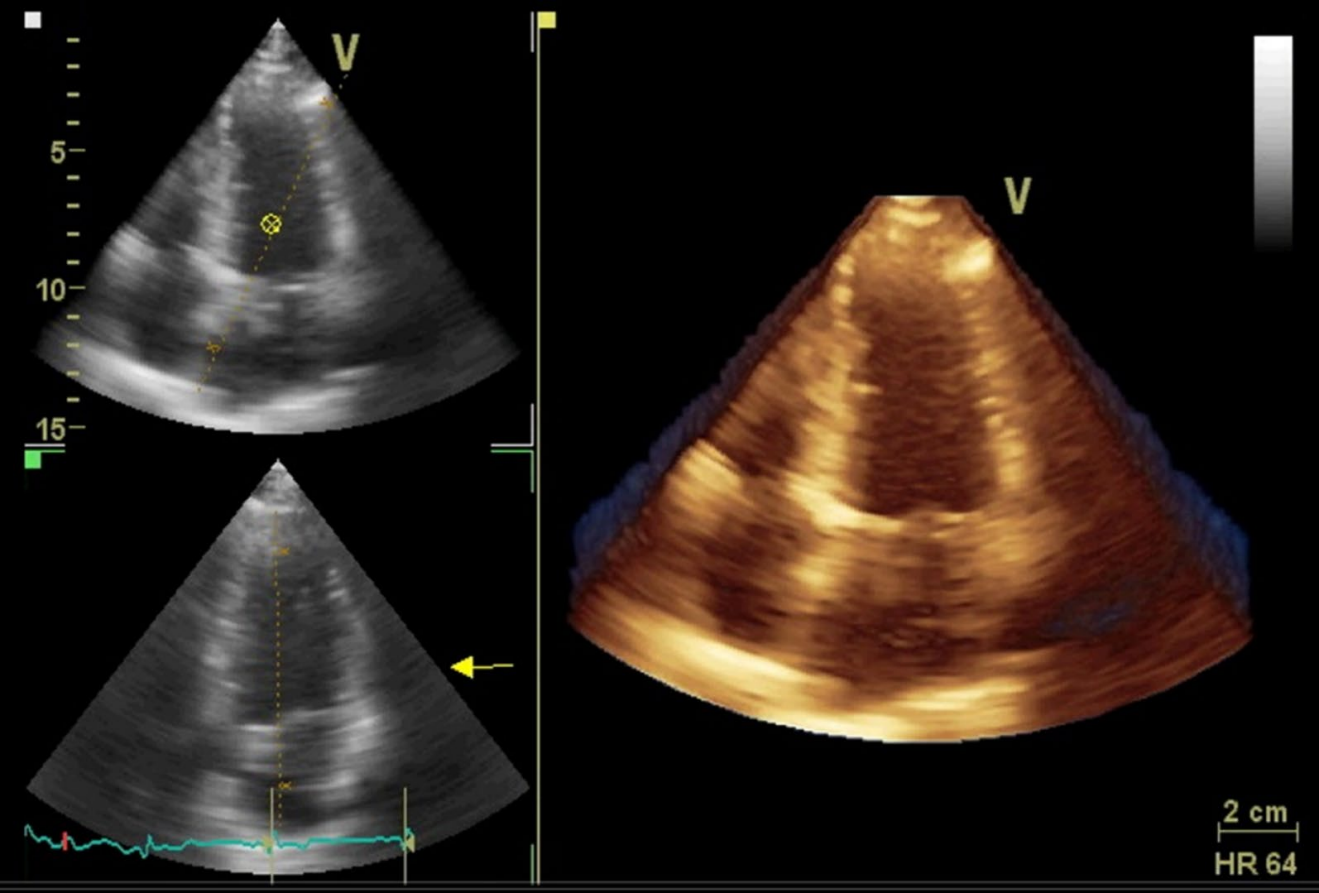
Three-dimensional full-volume echocardiography
